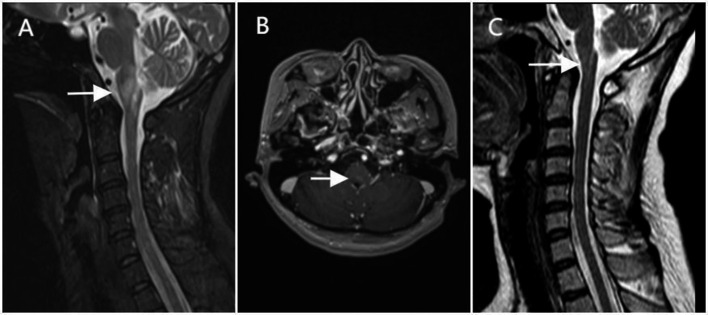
MRI findings of Case 2. A 41-year-old woman with area postrema syndrome (initial presentation in July 2023). (A) Sagittal T2-weighted image shows a hyperintense lesion (arrow) in the area postrema of the medulla oblongata. (B) Axial contrast-enhanced T1-weighted image demonstrates mild enhancement of the lesion (arrow) in the medulla oblongata. (C) Follow-up sagittal T2-weighted cervical spine image (September 2024) reveals reduction in the size of the lesion (arrow) compared with the initial presentation.
